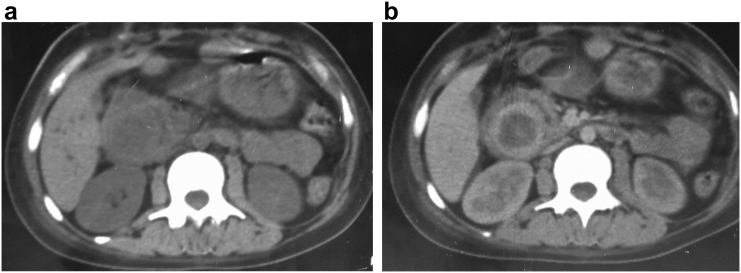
Computed tomography: axial CT scans of the abdomen before (a) and after contrast injection (b) shows a solid and hypodense mass with peripheric enhancement and central necrosis.
